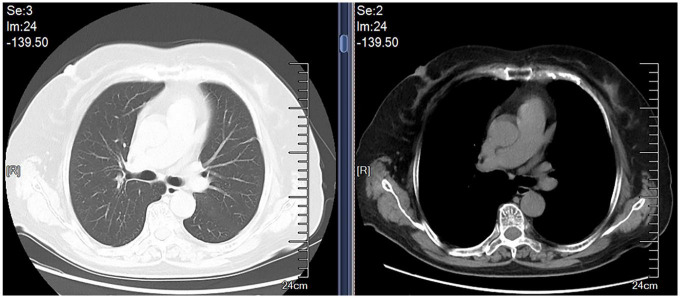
Follow-up chest CT showed a complete withdrawal of the left pleural effusion.
